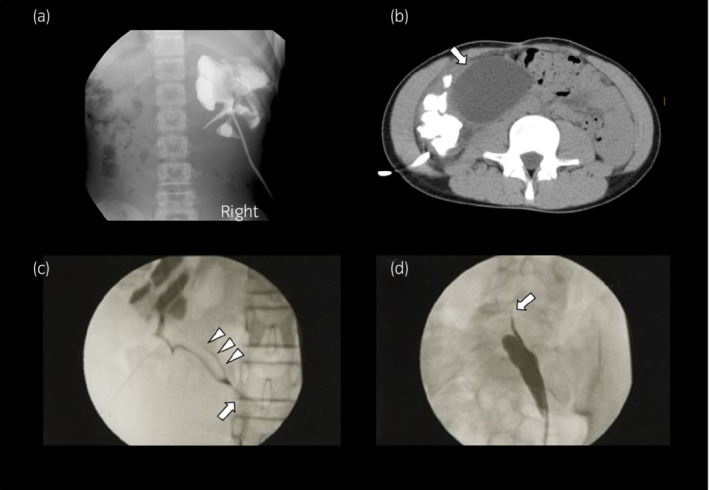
(a) Right antegrade pyelography after nephrostomy reveals shrinking of the renal pelvis and calyx; a right PUJO is suspected. (b) Plain CT performed immediately after right antegrade pyelography reveals a cystic lesion separating from the right pelvis (arrow). (c) Right retrograde pyelography reveals a pelvic filling defect due to a clot (arrowheads) and a PUJO (arrow). (d) Left retrograde pyelography reveals the blind end (arrow) of the ureter in the pelvis.
